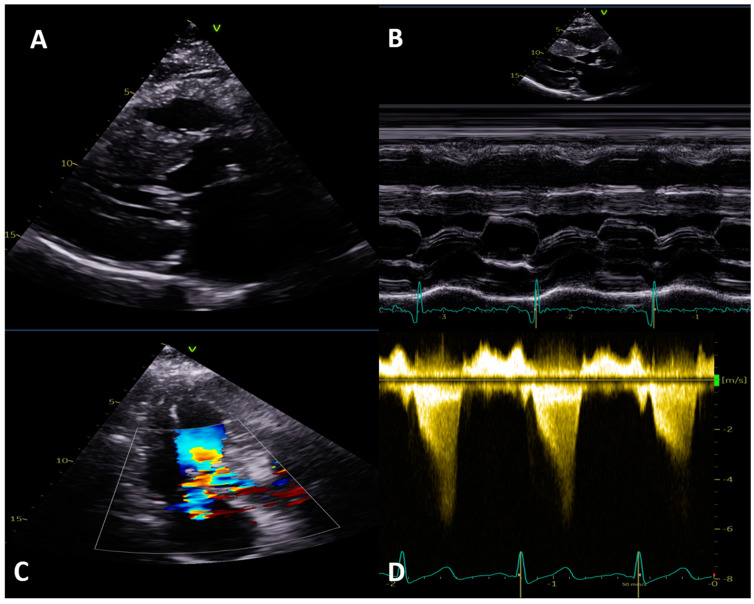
Left ventricular outflow tract obstruction (LVOT) in hypertrophic cardiomyopathy with transthoracic echocardiogram. (A,B) Systolic anterior movement of anterior mitral valve leaflet on parasternal long axis and M-mode. (C) Colour flow showing LVOT acceleration with associated posteriorly directed mitral regurgitation. (D) Continuous wave Doppler showing typical ‘dagger shape’ Doppler tracing indicating LVOT obstruction.
